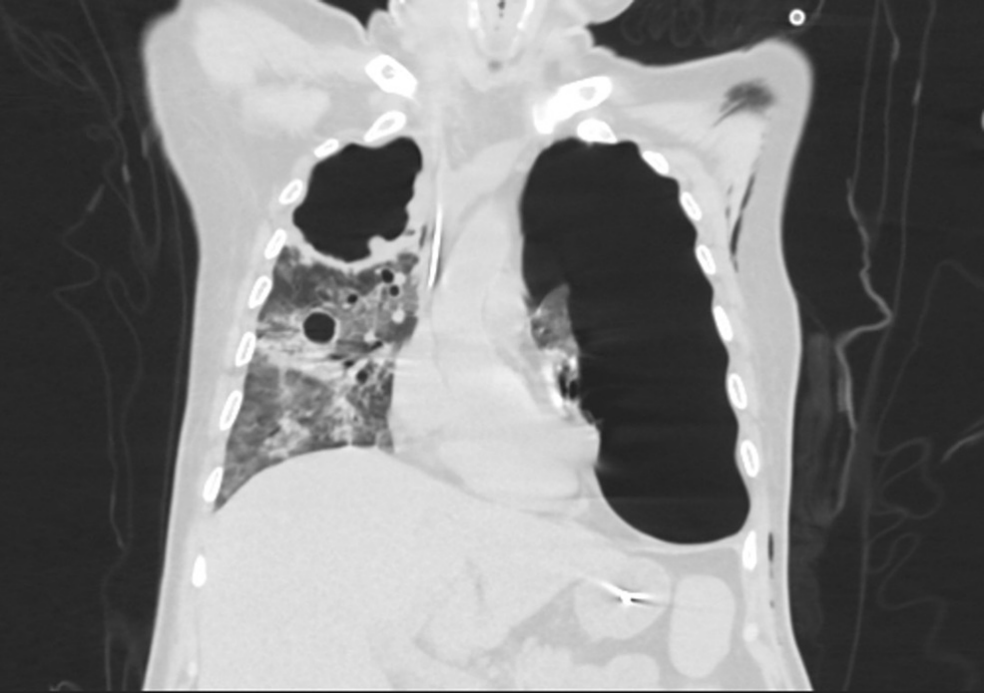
Selected coronal CT image showing a large right upper lobe cavity with an irregular nodular wall
